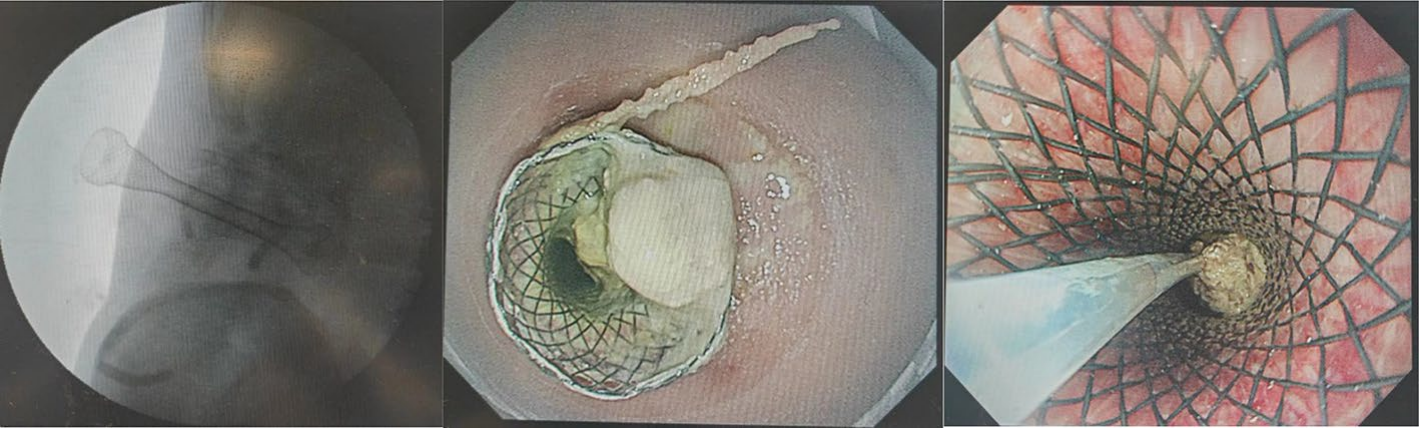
Percutaneous endoscopic necrosectomy (left: X-ray imaging of deployment of a covered self-expandable metal stent; middle: endoscopic insertion percutaneously; right: the removal of necrotic tissue through the stent).
